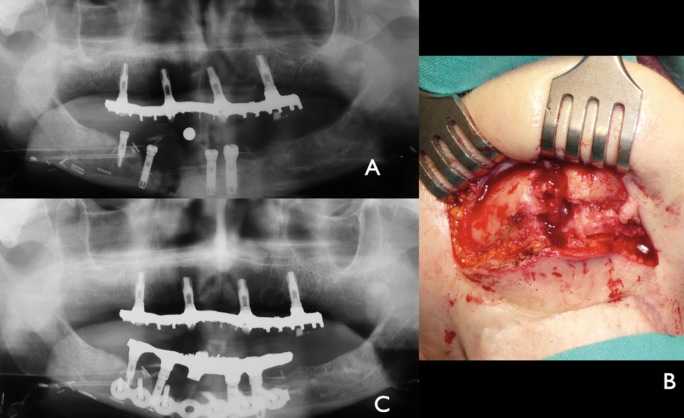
Case 1: A) Anterior mandibular fracture at the site of one of the implants with PI. B) Extraoral approach to expose mandibular fracture. C) Orthopantomography postoperative showing fracture reduction and stabilization by titanium reconstruction plate.
